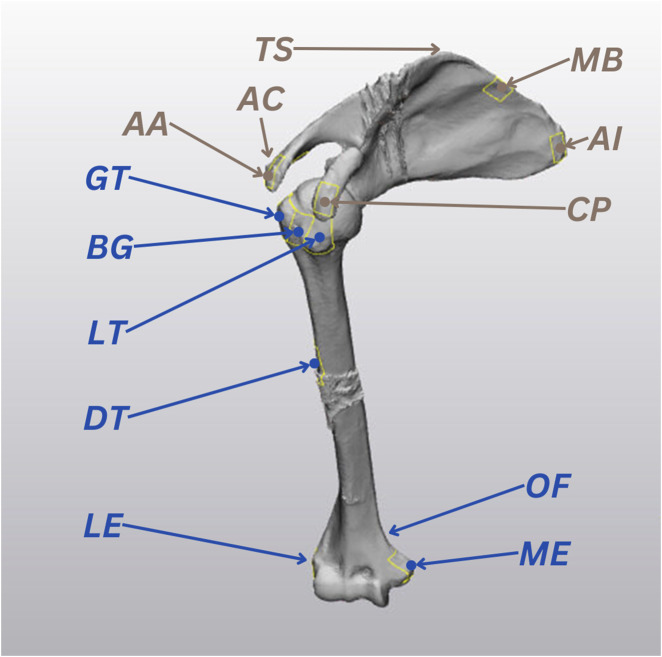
The 13 ultrasound (US) landmarks selected for the study, based on the International Society of Biomechanics recommendations (Wu et al., 2005). Humeral landmarks are highlighted in blue and scapular landmarks are represented in brown.
